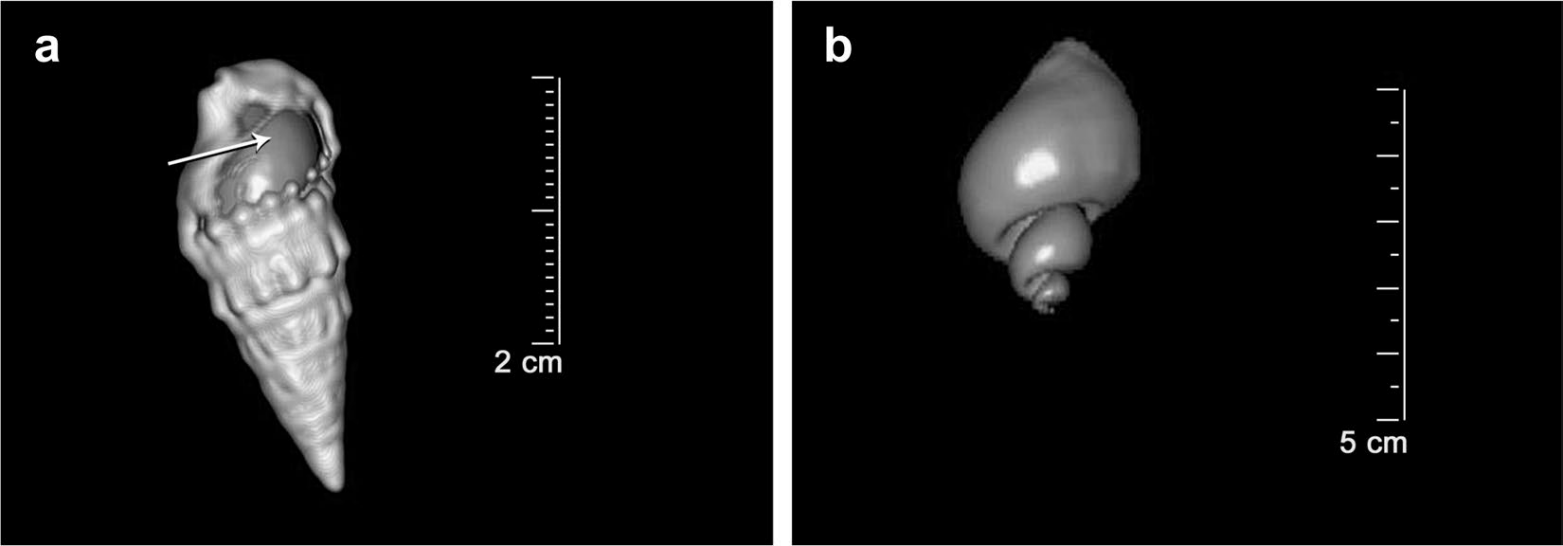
Three-dimensional images reconstructed by CT Viewer software of: (a) a *Cerithium atratum* shell showing the volume of air that fills the shell cavity (arrow) and (b) the air volume isolated from the shell cavity of *Stramonita haemastoma*.
